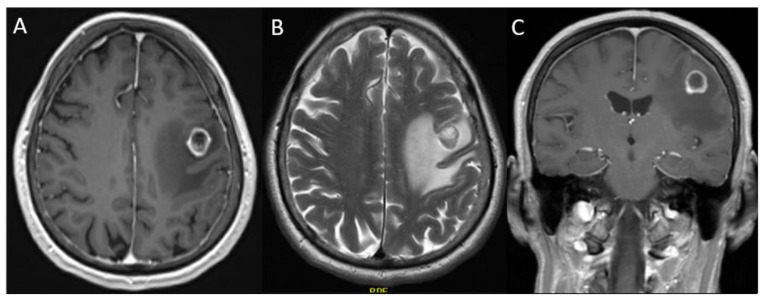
Preoperative MRIs. (A): Axial weighted T1 with contrast demonstrating a well circumscribed lesion in the left precentral gyrus with surrounding hypointense signal representing edema. (B): T2-Weitghed MRI with a hyperintense, ovoid, well-defined lesion within the left precentral gyrus. Vasogenic edema is visible in this image as a hyperintense signal around the mass. (C): Weighted coronal T1 with contrast demonstrating clear visualization of the lesions well-circumscribed margins.
